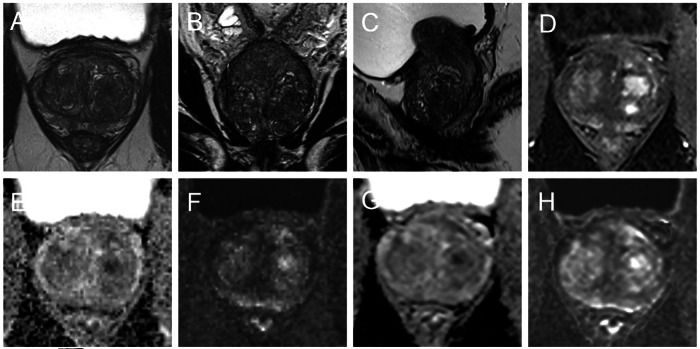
68 age, Status post positive TRUS biopsy (1/12 cores; left mid anterior; max. infiltration 40%; ISUP GG1; T1c, G2). PSA stable 6.4 ng/ml. Prostate volume 79 ml, 78 ml on prior MRI. PSA density 0.08 ng/ml/ml, stable 0.08 ng/ml/ml. Intravesical Prostate protusion (IPP): 23 mm. Follow-up MRI (A-H) Atypical hyperplasia at the apex and left anterior, no relevant progress. PI-RADS (v2.1): 3 PRECISE-Score (v2): 3-nonV MR Staging: cT2a.
